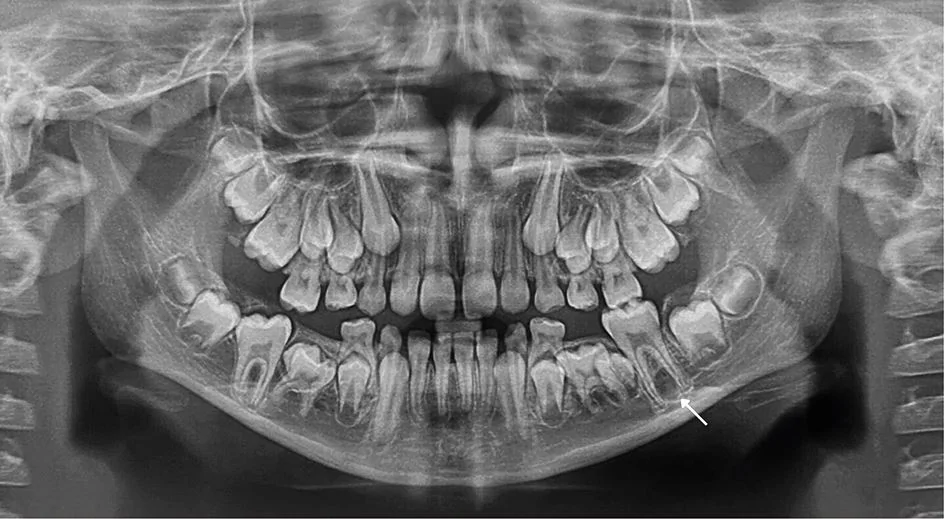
Panoramic radiograph of Case 2 (at ten years old) previously obtained, which shows a radiolucency in the interradicular region of tooth 36 (arrow)
